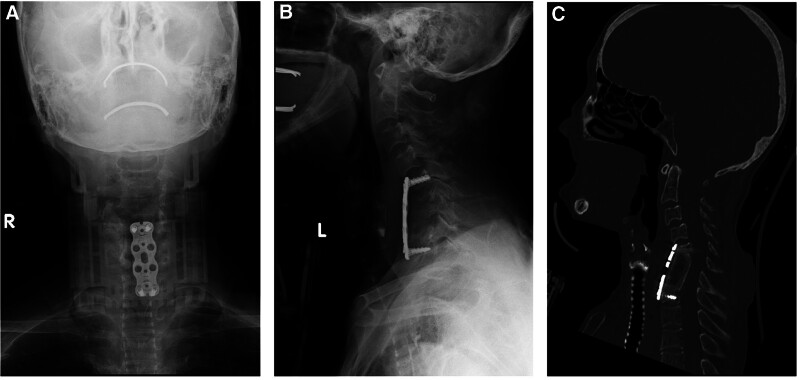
Cervical spine X-ray and CT after 1 week of anterior cervical corpectomy and fusion (ACCF). (A) The anteroposterior view; (B, C) the lateral view.
